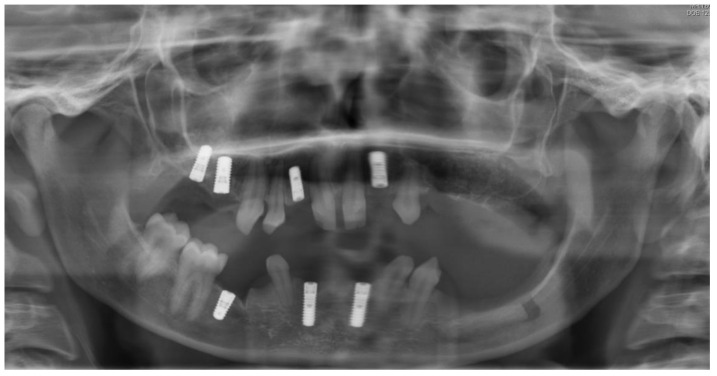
Panoramic radiography after bone graft, nerve lateralization, and placement of dental implants in edentulous sites with adequate bone volume.
